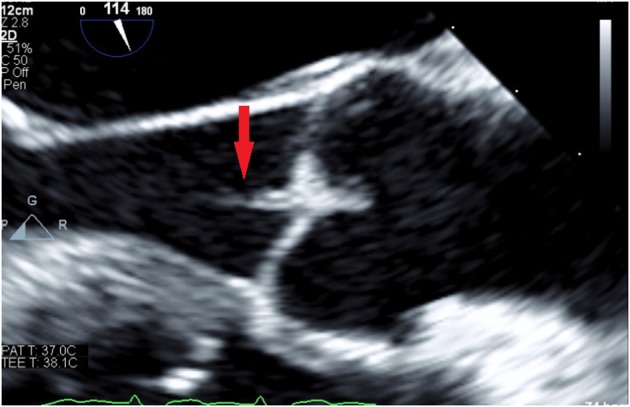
Transesophageal echocardiogram demonstrating small aortic valve vegetation (red arrow).
